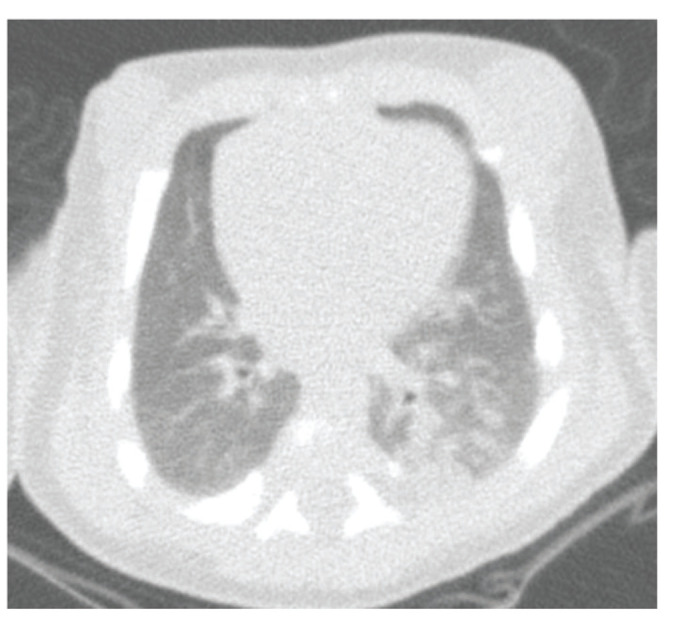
JCR chest computed tomography (case 3). Multiple pulmonary opacities and sparse focus of the consolidation of bilateral multifocal distribution, with peripheral predominance and greater extension in the left lower lobe.
